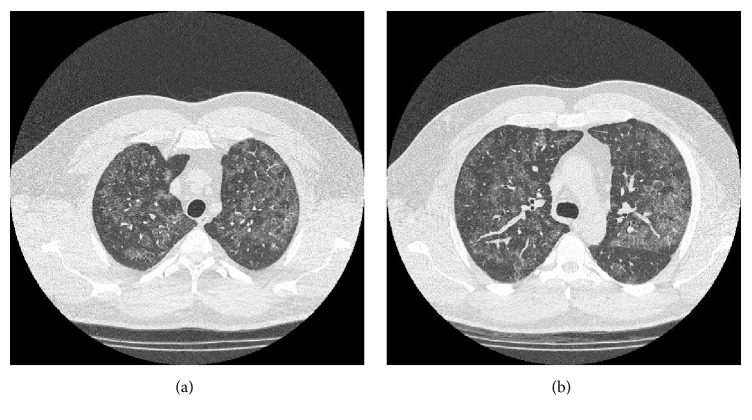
(a) and (b) Bilateral diffuse ground-glass opacities with thickened interlobular septae were seen in multiple sections of the lungs on HRCT.
